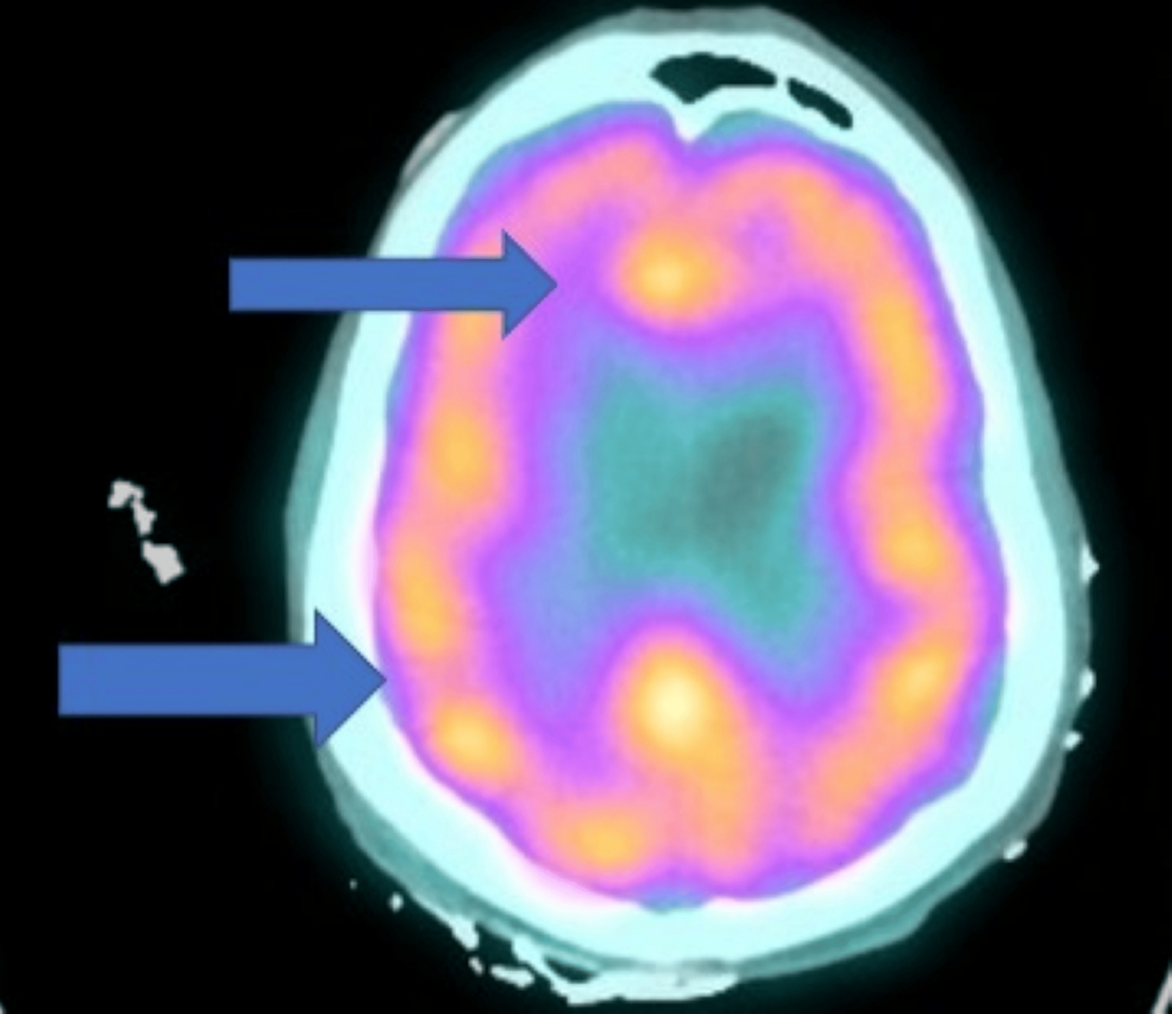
Brain single-photon emission computed tomography Brain single-photon emission computed tomography with markedly diminished activity in the anterior corpus callosum and right poster parietal-temporal lobes.
